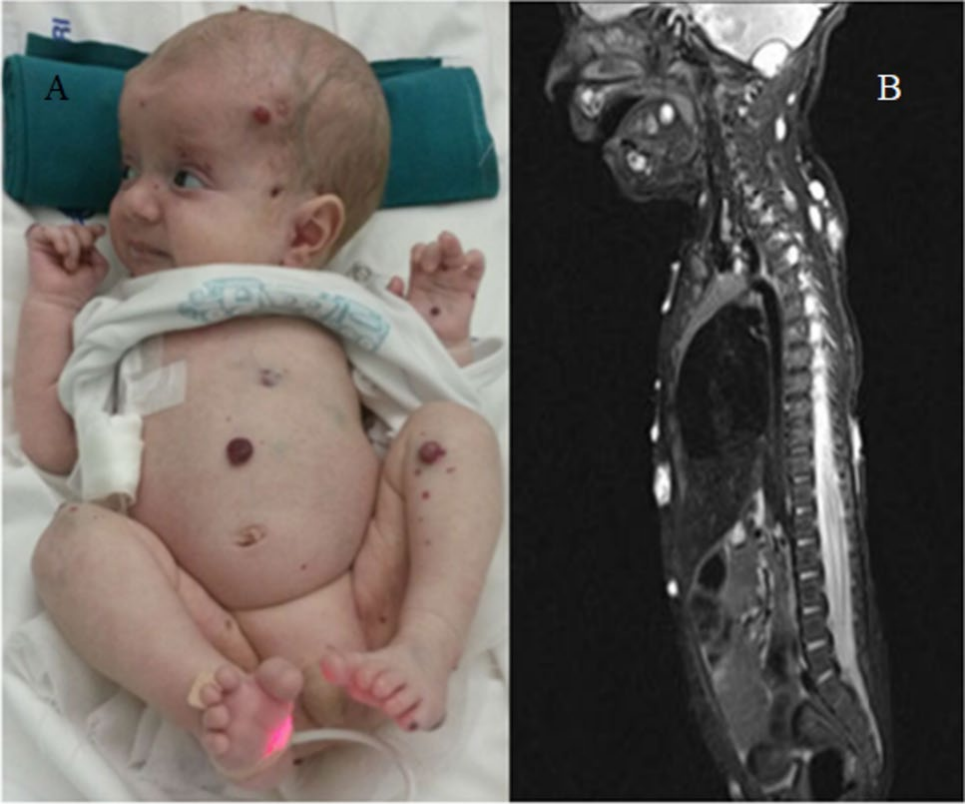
A Multifocal cutaneous red skin lesions of the face, trunk, abdomen, and limbs; B sagittal T2-weighted images (T2WI) showing subcutenous and subfascial lesions
